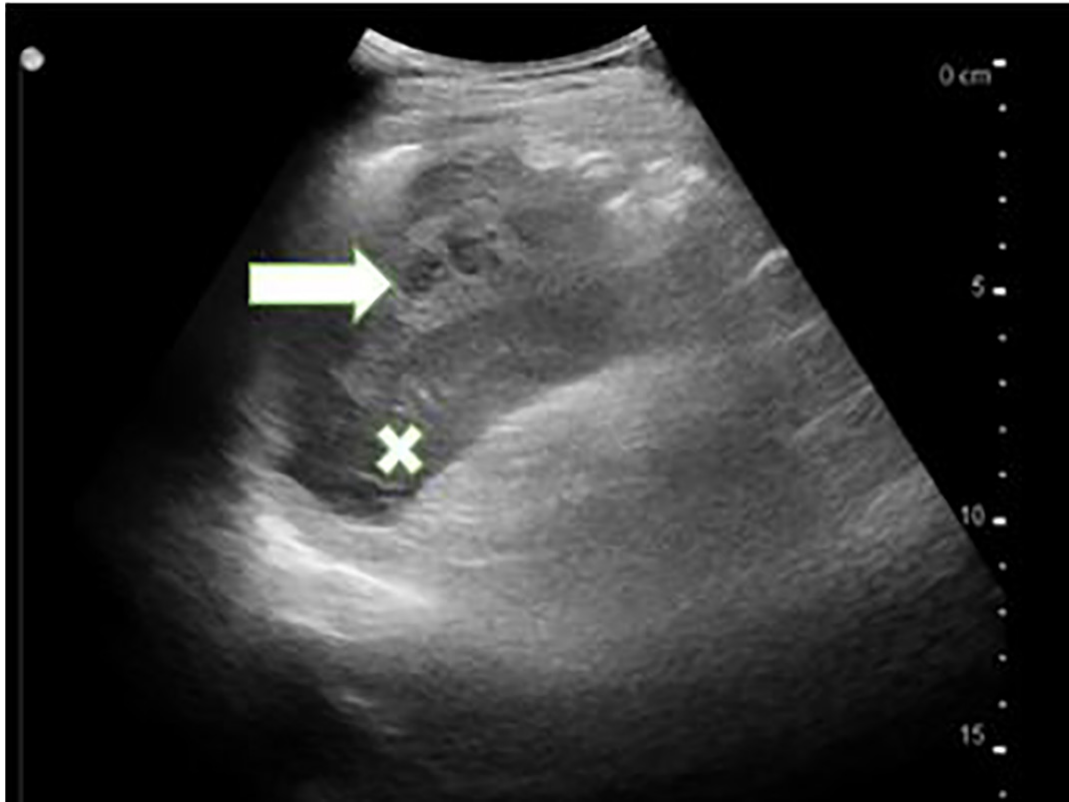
Point-of-care ultrasound of the spleen (X) with mixed hypoechoic densities within the splenic capsule (arrow).
